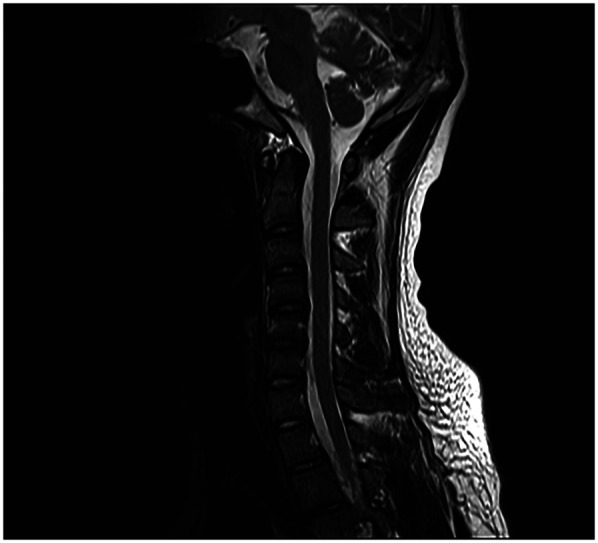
MRI of the left shoulder (WATER: BH Cor LAVA-flex + C). This MRI, performed 10 days post-surgery, shows no notable morphological abnormalities in the bilateral brachial plexus nerves. Both shoulder joints and their associated soft tissues also appeared morphologically normal, ruling out structural causes like masses or ruptures.
